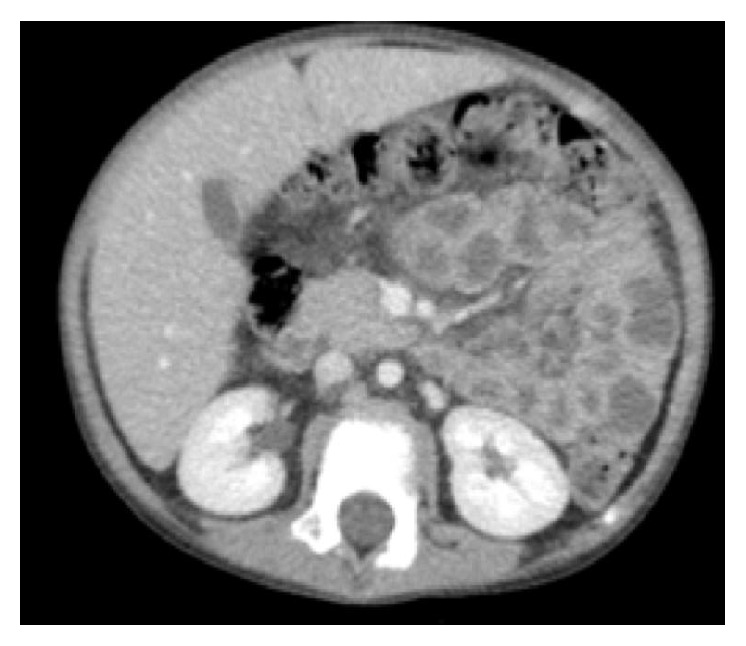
CT abdomen with contrast after induction therapy for ALL. Interval resolution of small hypodense subcortical lesions within both kidneys, consistent with favorable response to therapy of leukemic deposits in the kidney.
